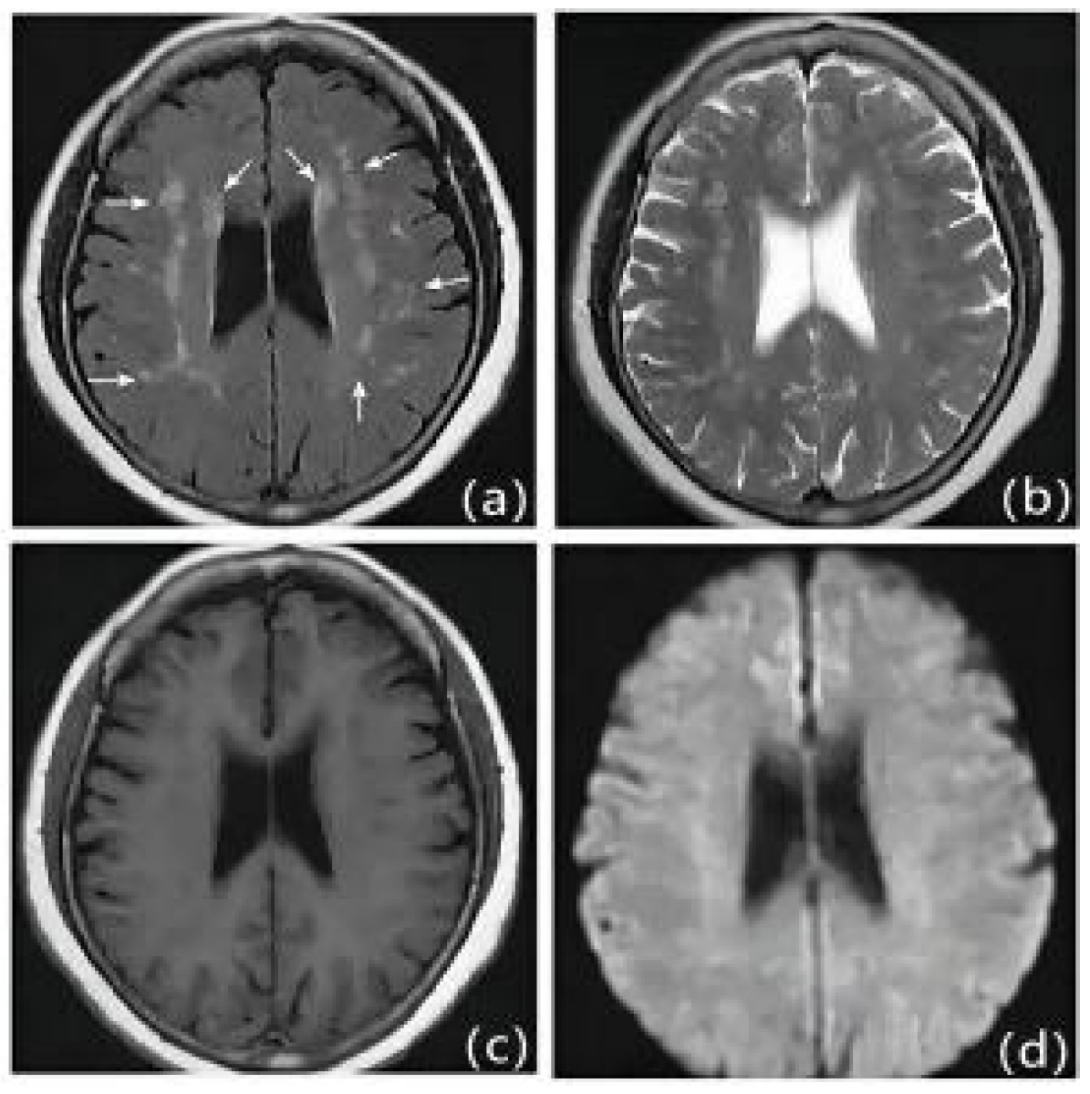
MRI presentation of LA. a: FLAIR, b: T1WI, c: T2WI, d: DWI.
